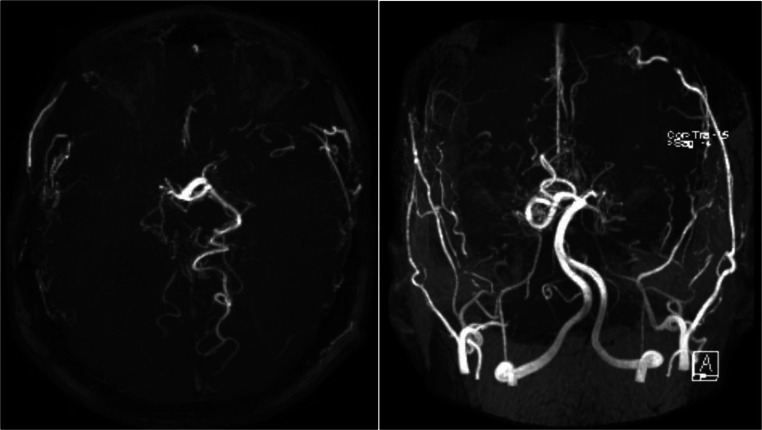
MRI angiography of case 1 showing bilateral internal carotid artery stenosis leading to Moyamoya diagnosis. This was prior to surgical revascularization
